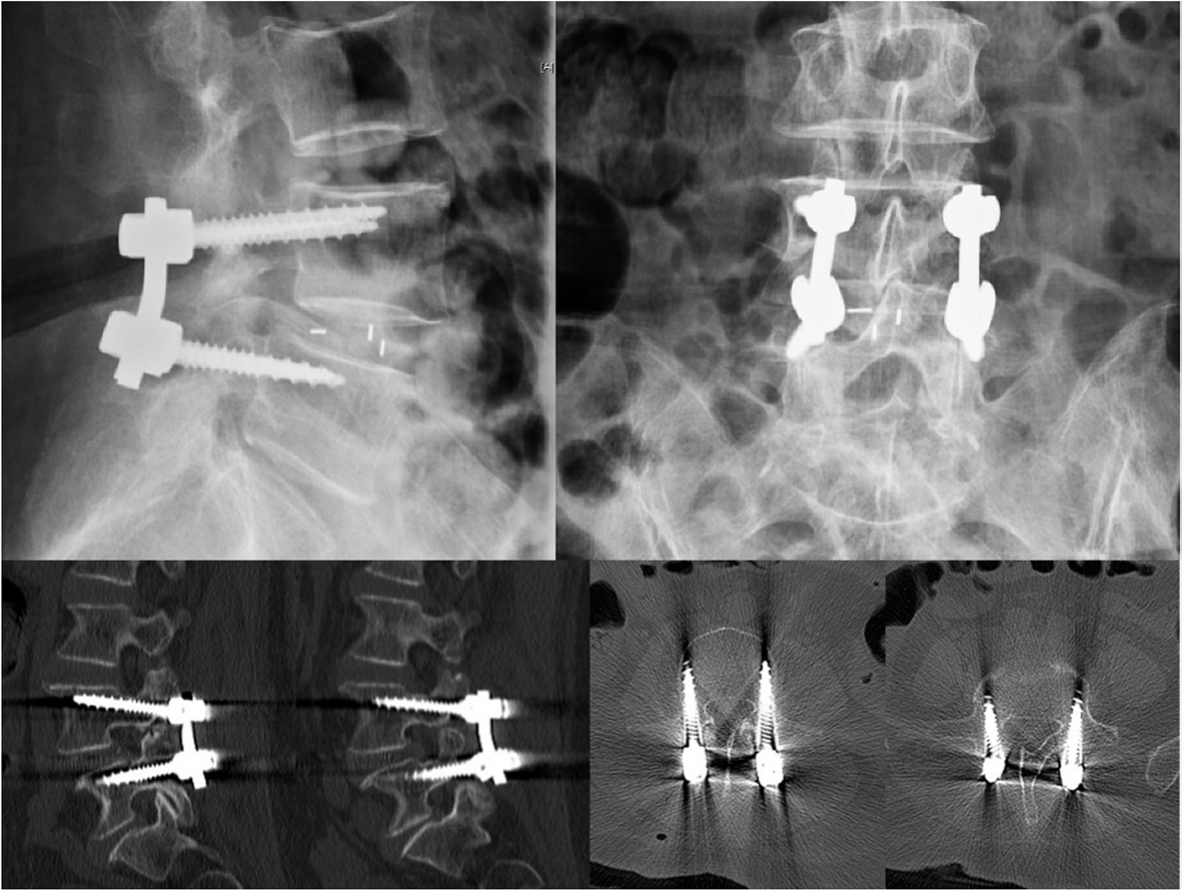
Postoperative reexamination of lumbar CT showed that all four screws reached grade 0 follow the score proposed by Ding et al. [12]
